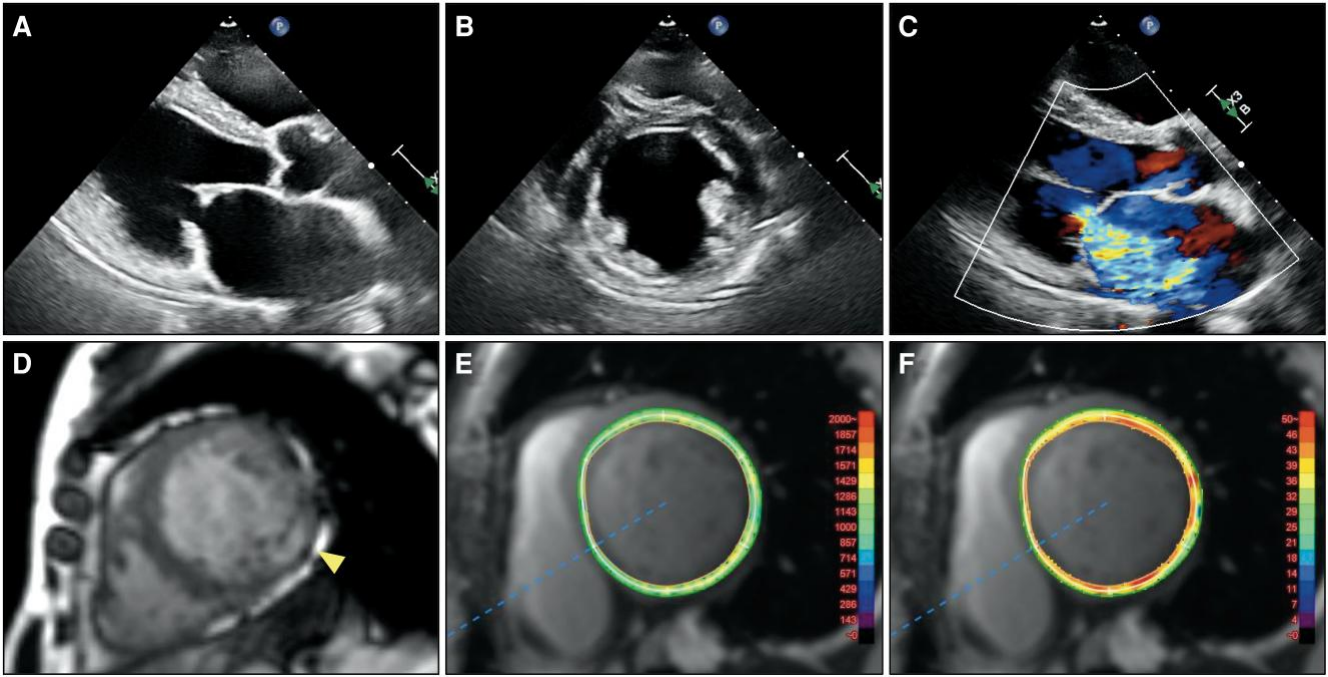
Multimodal imaging findings in 2023. (*A–C*) Transthoracic echocardiography revealing that the left ventricular wall motion was diffusely impaired in addition to the thinned inferior-to-inferolateral wall, and left ventricular ejection fraction was decreased to 42%. Mitral regurgitation became severe. Left ventricular hypertrophy was not identified. (*D*) On cardiac magnetic resonance imaging, transmural late gadolinium enhancement is observed in the thinned inferior-to-inferolateral wall (arrowhead). (*E* and *F*) T1 mapping analysis indicating that native T1 value and extracellular volume are diffusely elevated, including in non-late gadolinium enhancement areas. The mean native T1 value and extracellular volume are 1318 ms (normal: 1182 ± 24 ms) and 40.3% (normal: 23–28%), respectively.
